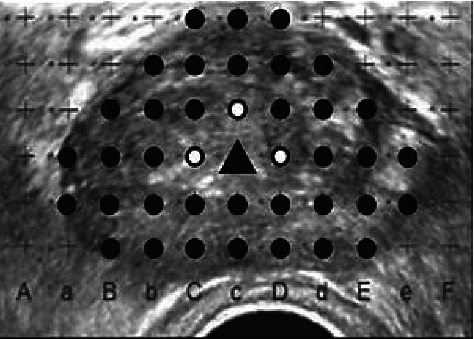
Transrectal ultrasound image at transperineal template saturation biopsy. Ultrasound image of the prostate showing the sites for sampling (black dots). Triangle shows urethra. White dots show sites where sampling was avoided.
